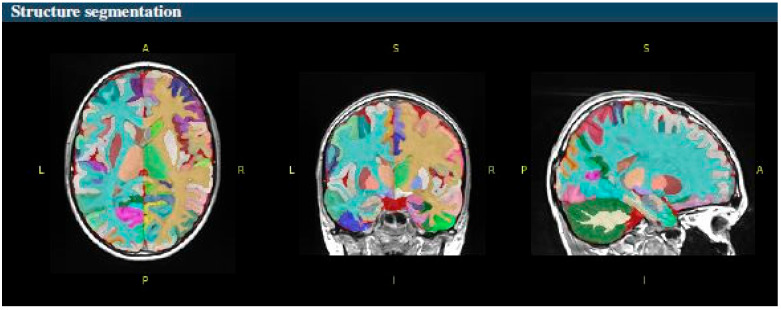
3D T1-weighted image structure segmentation in axial (left image), coronal (middle image), and sagittal plane (right image); A—anterior; P—posterior; R—-right; L—left; S—superior; I—inferior. Color maps for brain segmentation are used from Mindboggle software https://github.com/nipy/mindboggle/blob/master/mindboggle/mio/colors.py (accessed on 20 October 2024).
